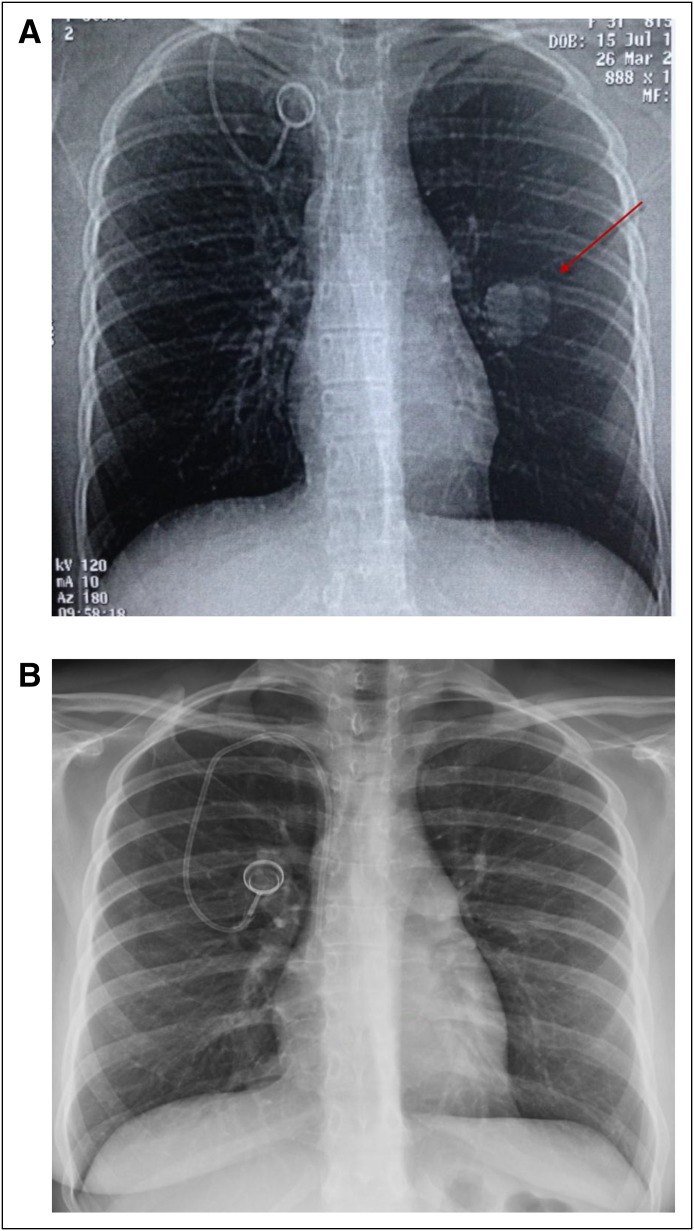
(A) Pre- (March 26, 2012; red arrow indicates tumor) and (B) post-treatment (July 17, 2013) chest x-rays. Patient remains in remission.
